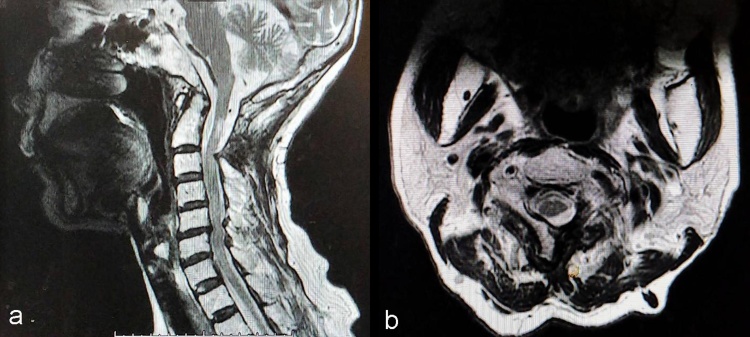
Cervical spine MRI nine months after the operation, there was no sign of recurrence.
